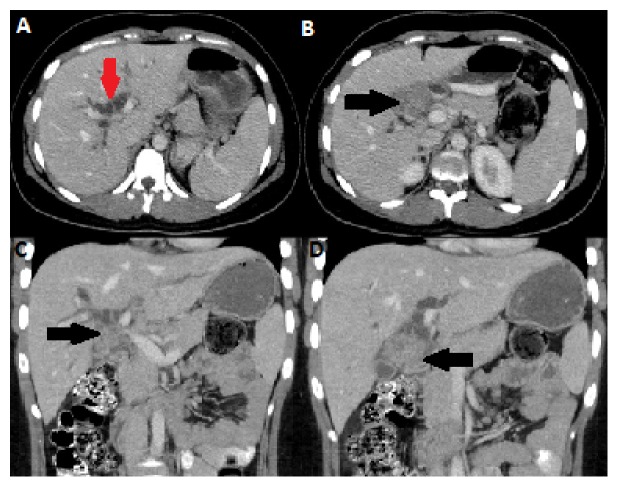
Enhanced CT scan of the abdomen axial (A, B) and coronal (C, D) images demonstrate an enhancing mass in the region of the porta hepatis (black arrow) with biliary duct dilatation (red arrow).
